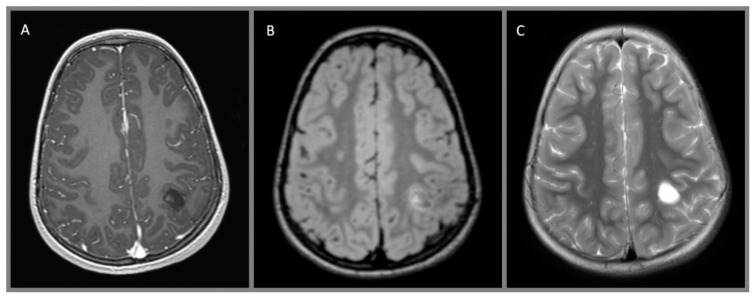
Dysembryoplastic neuroepithelial tumor, WHO I: (A) axial T1 MPRAGE with contrast, (B) axial T2 FLAIR with contrast, and (C) axial T2 without contrast demonstrate a small cystic lesion abutting the central sulcus in the left hemisphere. There is a small nodule and rim of enhancement at the medial aspect of the tumor.
